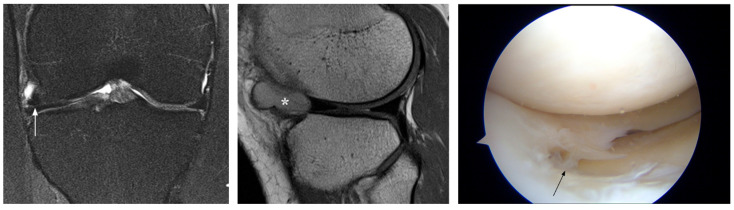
Equivocal false-negative MRI diagnosis (patient 27). MRI shows questionable grade 2 signal change at the anterior horn of the lateral meniscus (arrow) with a parameniscal cyst (*), but arthroscopy confirmed a degenerative tear at this location (arrow).
